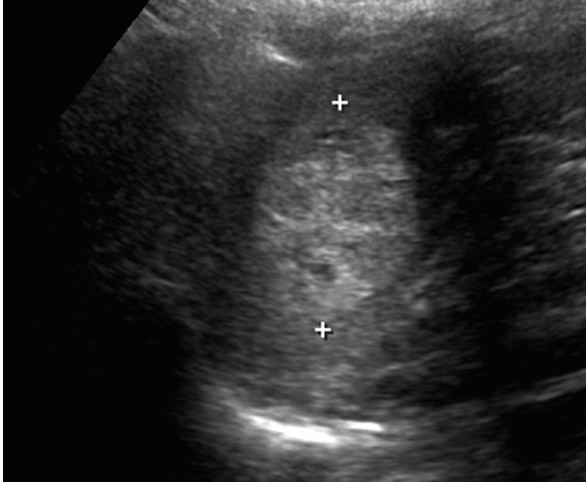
Ultrasound of the spleen demonstrates a well-defined hyperechoic lesion.
